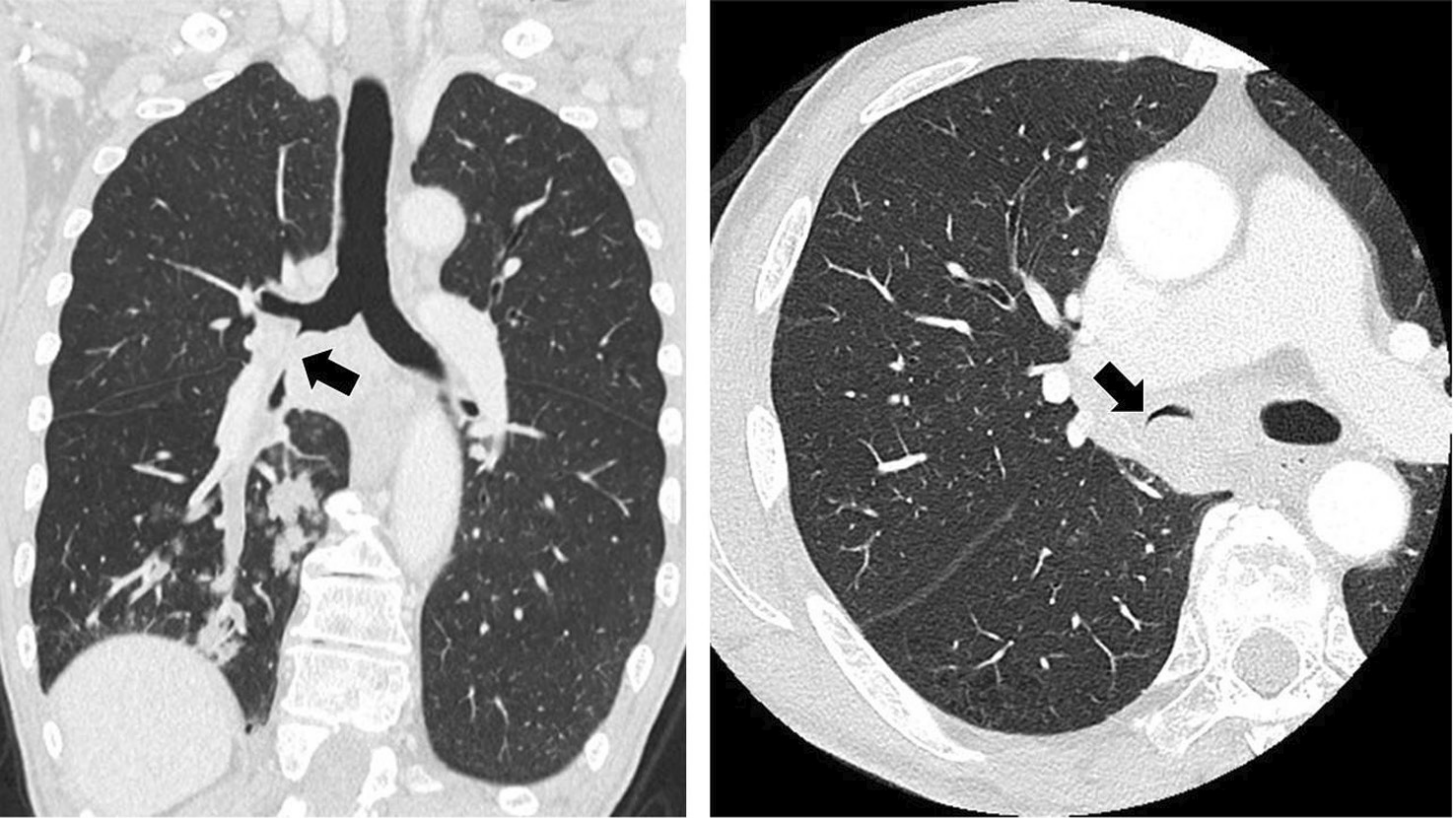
Chest computed tomography on admission. Each image displays a mass lesion in the right lower lung field and enlarged lymph nodes below the tracheal bifurcation. The arrow indicates stenosis of the right middle bronchus.
